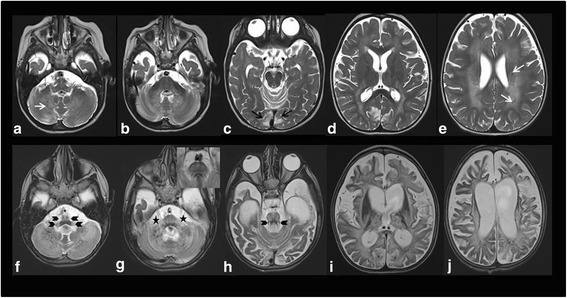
MRI in patient B. TOP, Axial T2WI. performed at 1 year and 9 months revealed diffuse hyperintensity in deep cerebellar white matter, middle cerebellar peduncles, brainstem and bi-hemispheric white matter (a, b, c, d). The signal is inhomogeneous for the presence of multiple focal marked hypointensities due to calcifications (e, arrows). BOTTOM, Axial T2WI. performed at 3 years and 10 months showed a dramatic extension of the diffuse signal abnormalities in both hemispheres with full involvement of the posterior arm of the internal capsules, external capsules, U fibers and thalami, with relative sparing of the putamina (h-j). The signal abnormalities extension was also evident in cerebellar white matter, cerebellar peduncles and brainstem (f, g). Bilateral symmetric hyperintensities in the bulbar pyramids and bulbar lateral regions (arrowheads in f), in the superior cerebellar peduncles and in the arciform fibers of their decussation (arrowheads in H) were more evident. The transverse fibers of the pons were prominent and hyperintense (insert in g). Both V cranial nerves appeared swollen and hyperintense (stars in g), as well as the optic chiasm. Calcarine cortex showed a gliotic hyperintensity (black arrows in c). A huge diffuse cerebral atrophy with ventricles and sulci dilatation associated with pronounced cortical thickness was also observed
